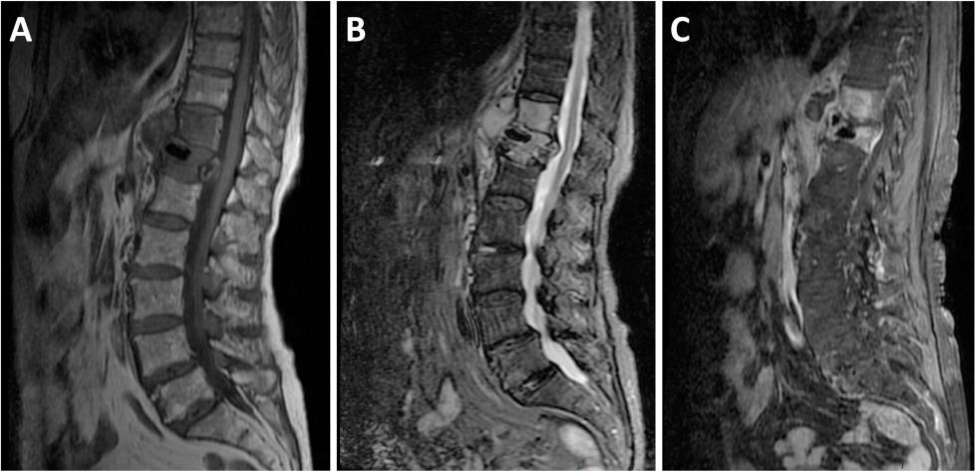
Sagittal Magnetic Resonance Imaging of the Lumbar Spine. A: T1-weighted non-contrast-enhanced. B: Short tau inversion recovery (STIR). C: T1-weighted fat-suppressed with Gadolinium-based contrast media.
